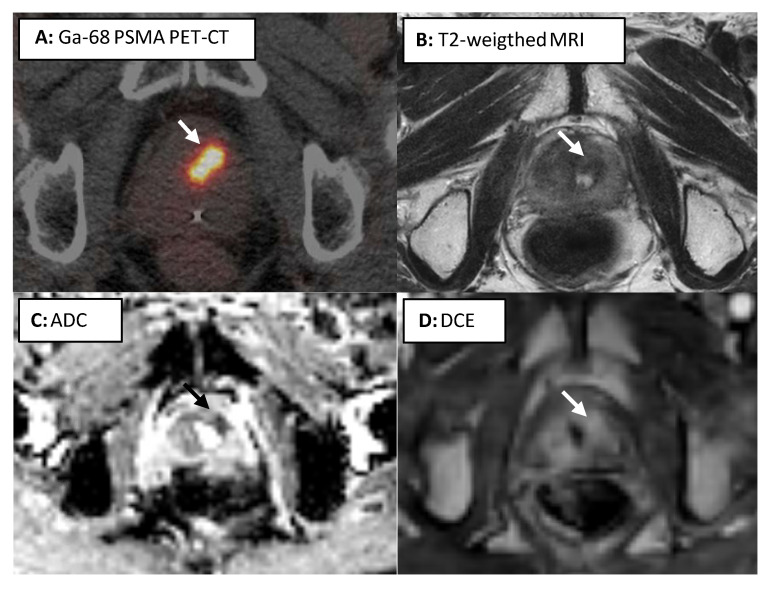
Example of an 82-year old patient who underwent external beam radiotherapy in 2010 with a total dose of 78 Gy in 39 fractions for a cT1cNxMx adenocarcinoma of the prostate, Gleason 3 + 4 = 7, PSA 8.8 ng/mL. He achieved a PSA nadir of 0.4 ng/mL in March 2013. In 2016, his PSA had risen to 3.3 ng/mL. A Gallium (Ga)-68 PSMA PET/CT scan showed a recurrence in the left mid-glandular anterior part of the prostate (Picture A, central activity due to urine in the urethra). This was verified on the T2-weighted image (low signal, picture B). Diffusion restriction was seen on the apparent diffusion coefficient (ADC) map (C) and contrast enhancement was seen on the dynamic contrast enhanced (DCE) series (D). He underwent MRI-targeted biopsies of this lesion which showed a recurrence in 7% of the biopsy volume in 1/5 cores, Gleason 3 + 4 = 7. He successfully underwent MRI-guided focal salvage HDR brachytherapy in September 2016. His PSA reached a nadir of <0.1 ng/mL, and slowly started to rise after 2 years, with a peak after 4 years followed by a decrease. No further diagnostics have been performed as of yet. Apart from transient urinary incontinence, no toxicity was reported.
